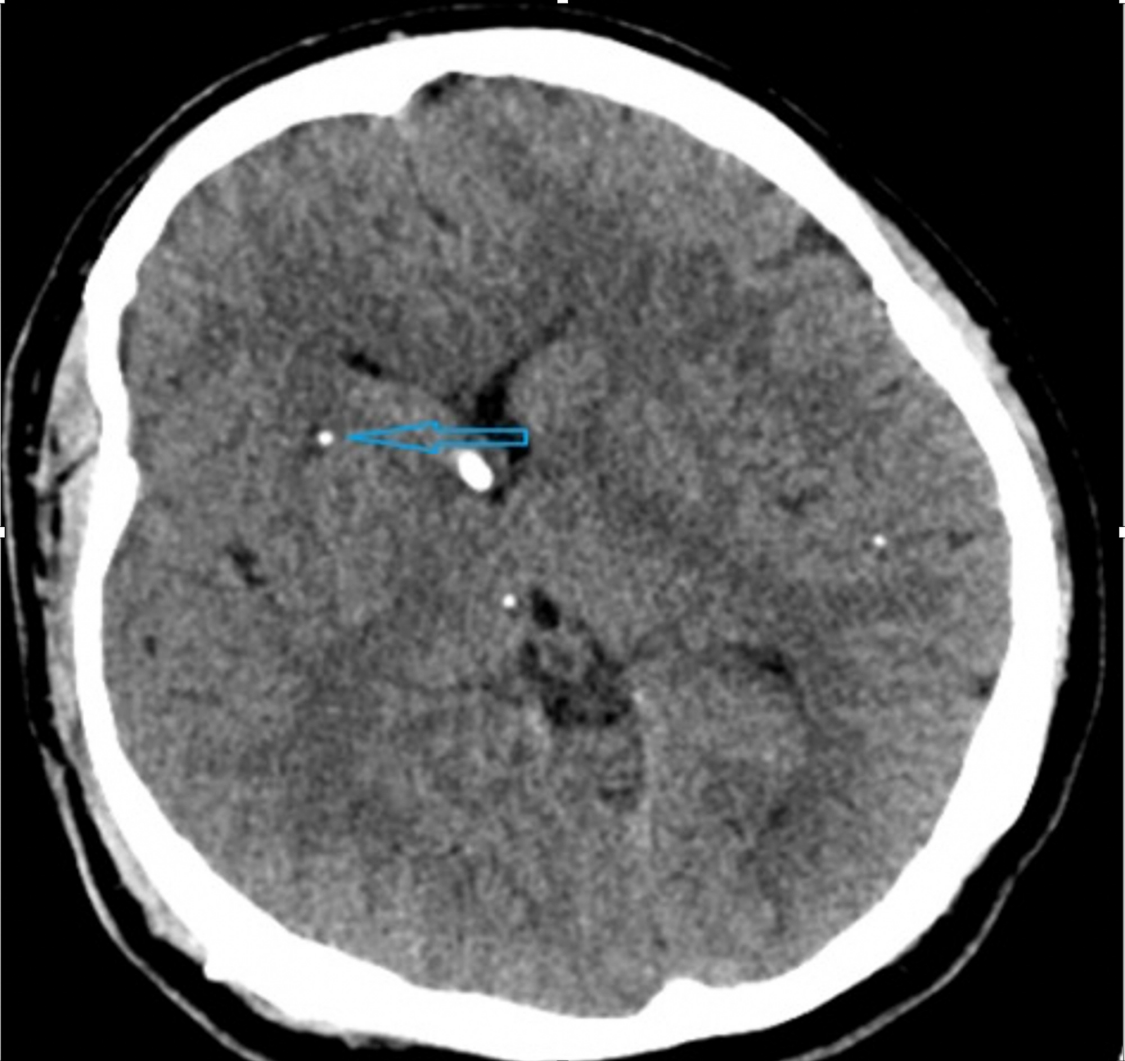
CT of the head showing calcifications (blue arrow).
